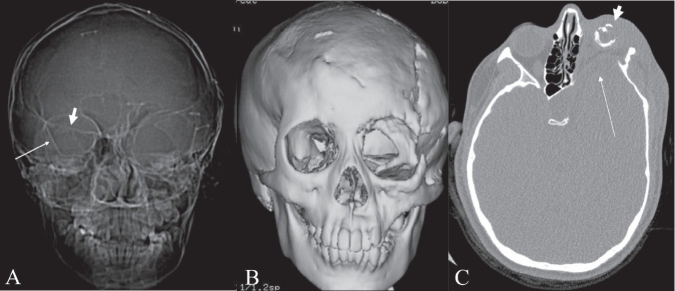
Bare orbit sign. Frontal CT scan scannogram of the head (A) shows a bare and large orbit on the left, with absence of the greater wing of the left sphenoid bone, in this patient with neurofibromatosis type 1. Note the normal lesser (thick small arrow) and greater (thin long arrow) wings of the sphenoid on the right side. Frontal surface-shaded display, 3D view of the skull (B) shows a large left orbit with dysplasia of the sphenoid wing. Axial CT scan image (C) shows anterior herniation of the left temporal lobe (thin long arrow) because of the dysplastic sphenoid bone. The left globe is small and calcified (phthisis bulbi) (thick short arrow) due to plexiform neurofibromatosis of the left orbit
